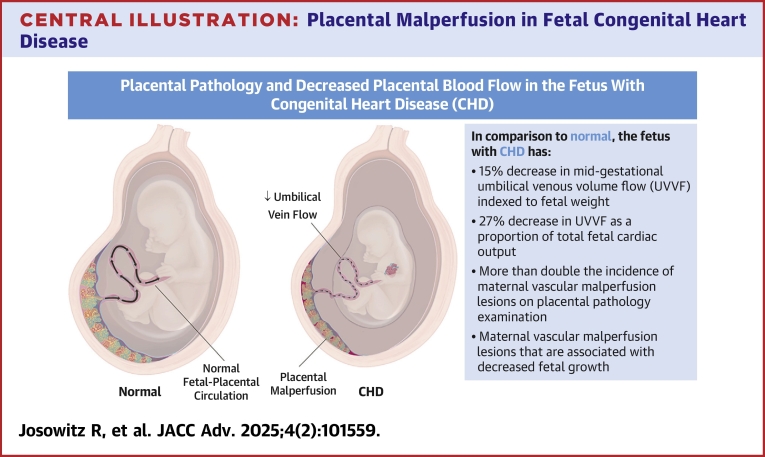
Placental Malperfusion in Fetal Congenital Heart Disease Fetuses with CHD have decreased blood flow from the placenta to fetus through the umbilical vein, as measured in second trimester fetal echocardiograms by umbilical venous volume flow. In the setting of unchanged cardiac output, decreased umbilical flow is suggestive of increased placental resistance. These fetuses have a higher incidence of placental malperfusion lesions at birth. Placental maternal vascular malperfusion lesions, in particular, are associated with decreased fetal growth in CHD. Abbreviation as in Figure 1.
